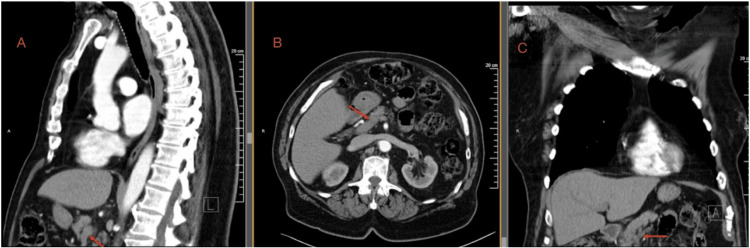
Follow-up contrast-enhanced computed tomography demonstrating the resolution of splanchnic venous thrombosis (red arrows) after anticoagulation Multiplanar contrast-enhanced computed tomography of the abdomen obtained three months after the initiation of apixaban therapy demonstrating the complete radiologic resolution of previously identified superior mesenteric and splenic vein thrombi. (A) Sagittal reconstruction demonstrating the normal opacification of the mesenteric venous system without residual filling defect. (B) Axial image demonstrating the patency of the superior mesenteric vein at the prior site of thrombosis (arrow). (C) Coronal reconstruction confirming the restoration of normal venous flow within the mesenteric venous confluence.
